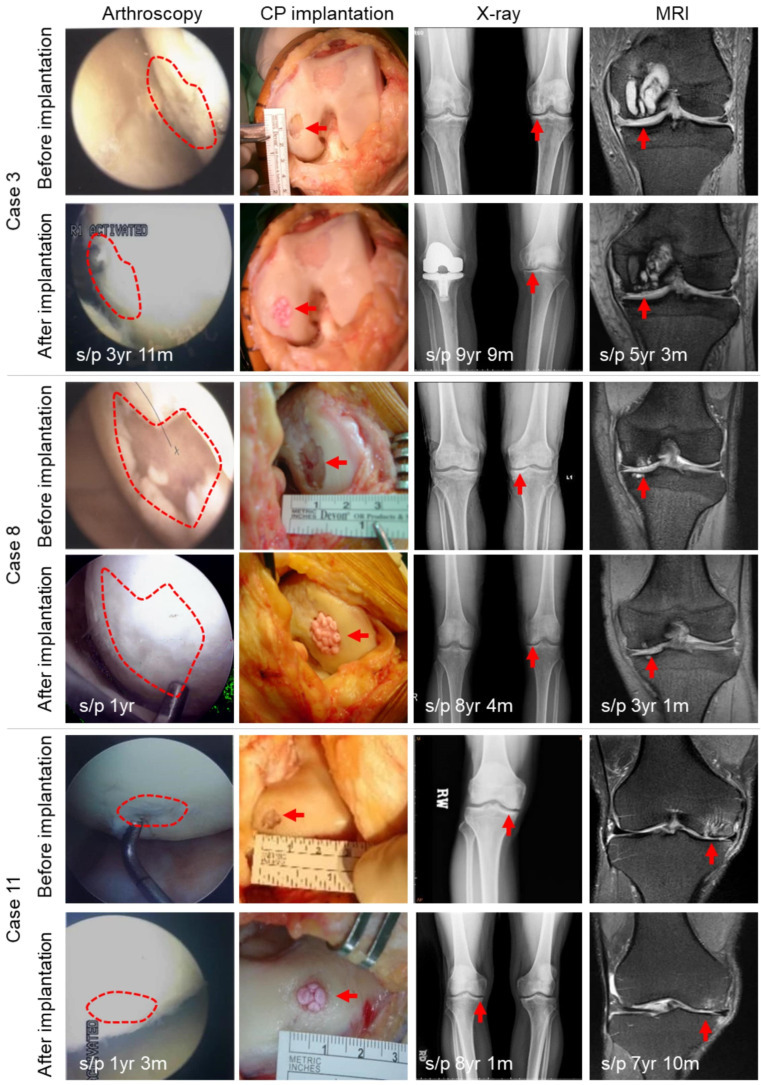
Assessments of the cartilage lesion and recovery before and after the CP implantation. Cartilage lesion as seen via arthroscopy, knee arthrotomy, X-ray, and MRI before and after the CP implantation for three representative patients (Cases 3, 8, and 11). The implanted CP tissue was softer than the native cartilage tissue on palpation with a probe. The defect sites are circled by the red dashed lines in the arthroscopy images and indicated by the red arrows on the other three types of images.
